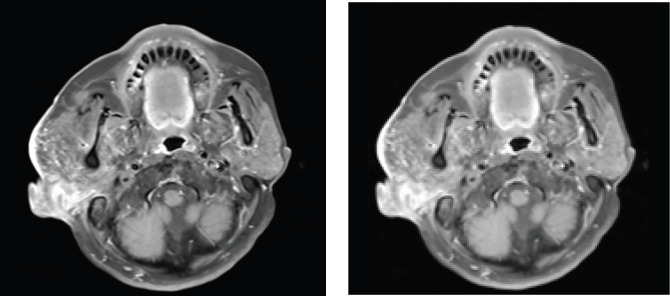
Result of maxillofacial MRI: Right external ear canal obstruction, size 37 × 31 mm, invasion of parotid gland, inner ear canal, with nearby lymph node metastasis.
